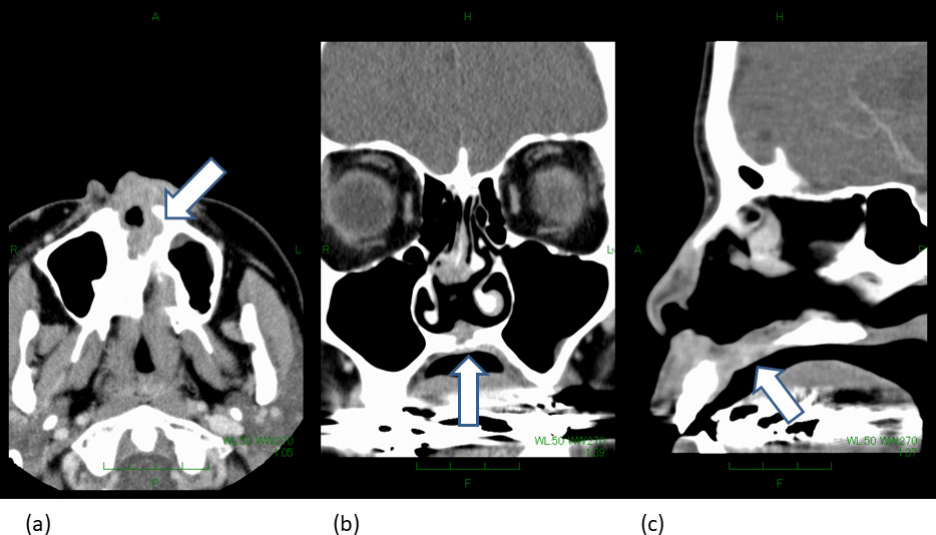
The figure shows pretreatment computed tomography of the patient with nasal vestibule cancer. (a), (b), and (c) represents the axial, coronal, and sagittal image, respectively. The white arrow represents a part where the tumor involves the bony structure of the hard palate.
